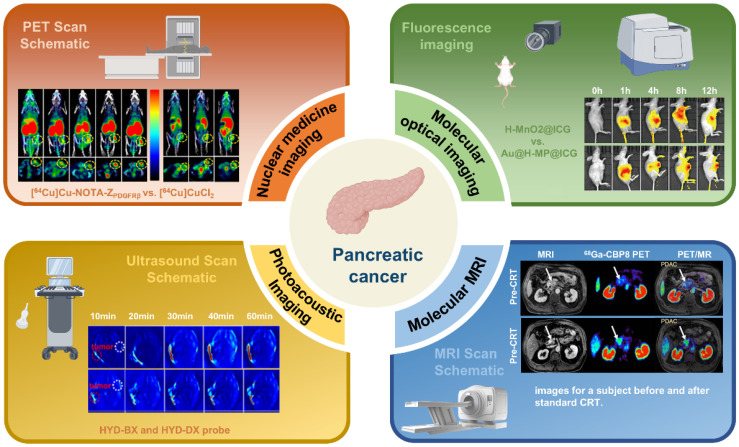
Exploring molecular imaging techniques for pancreatic cancer. This review summarizes recent advances in molecular imaging for pancreatic cancer, covering major technological platforms including nuclear medicine imaging, molecular optical imaging, photoacoustic imaging, and molecular MRI. *PET*, positron-emission tomography; *MRI*, magnetic resonance imaging; *CRT*, chemoradiotherapy. Adapted with permission from refs [15,16,17] (Copyright 2024–2025, American Chemical Society) and ref. [18] (available under a CC-BY 4.0 license, Copyright 2024).
